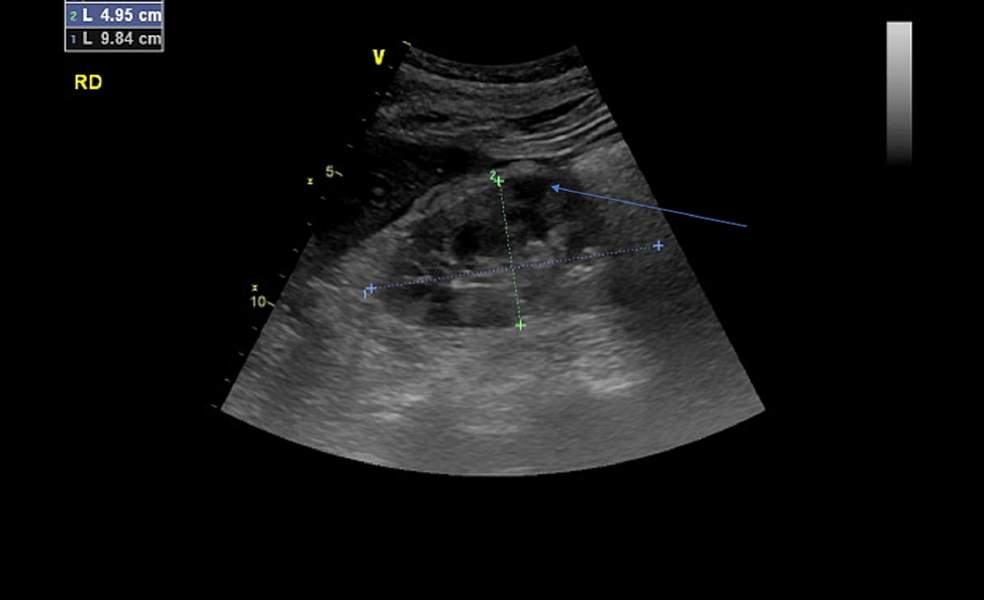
Duplex Doppler ultrasound showed aspects of multifocal cortical renal infarctions in the right kidney (arrow)
